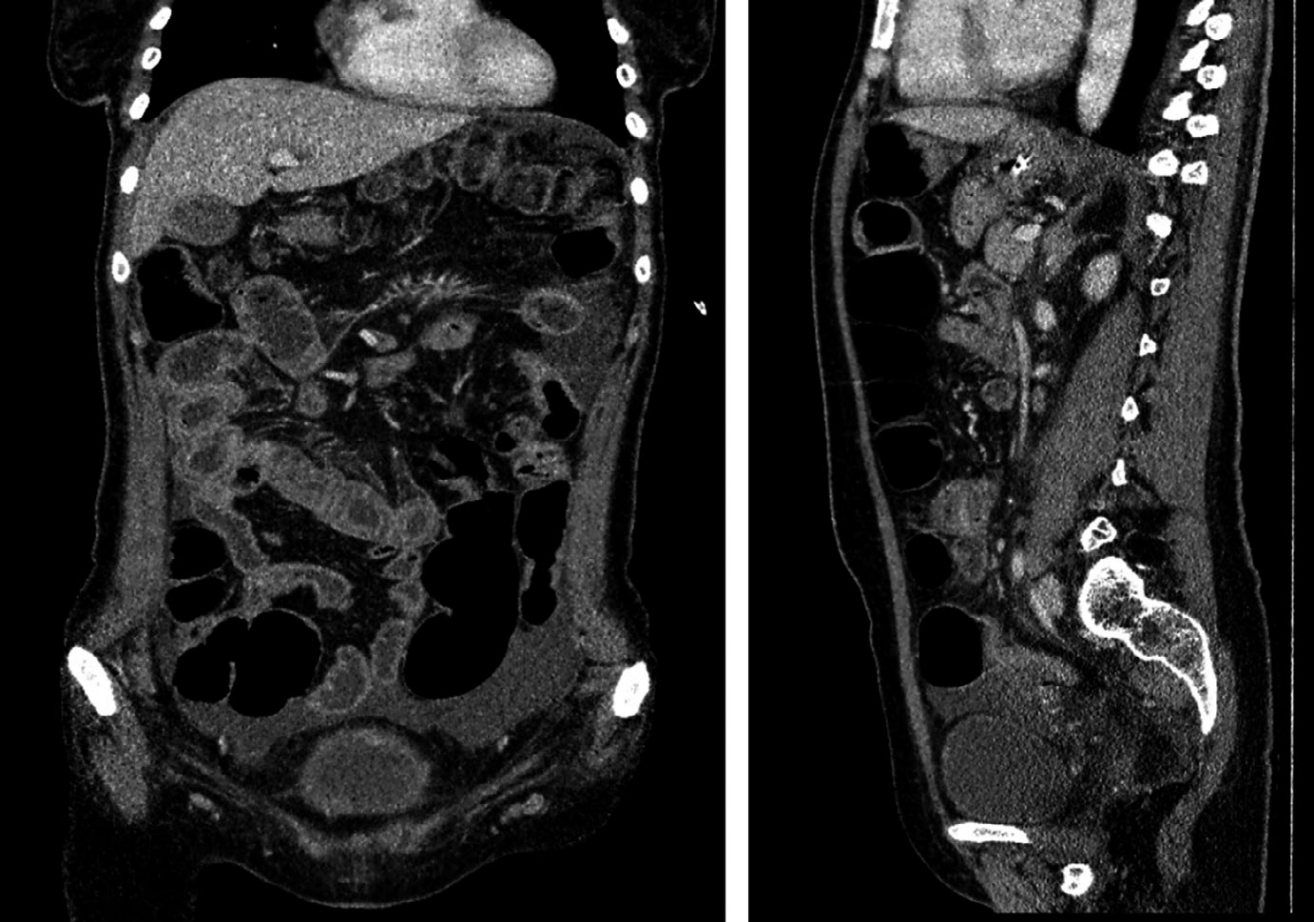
Abdominal CT scan views showing marked dilatation of small bowel loops and small intestinal wall thickening
